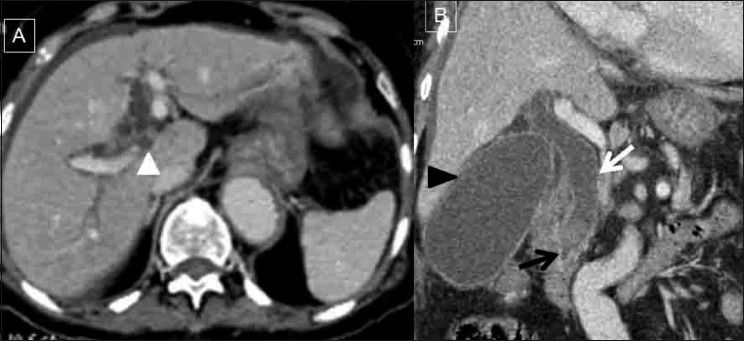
Axial (A) and coronal reformatted (B) contrastenhanced CT scans of the abdomen show an impacted sludge ball (black arrow in B) at the terminal common bile duct (CBD) leading to the proximal CBD and intrahepatic biliary duct dilatation (white arrowhead in A). Mild pericholecystic fluid and gall bladder wall thickening (black arrowhead in B) suggest acute cholecystitis
